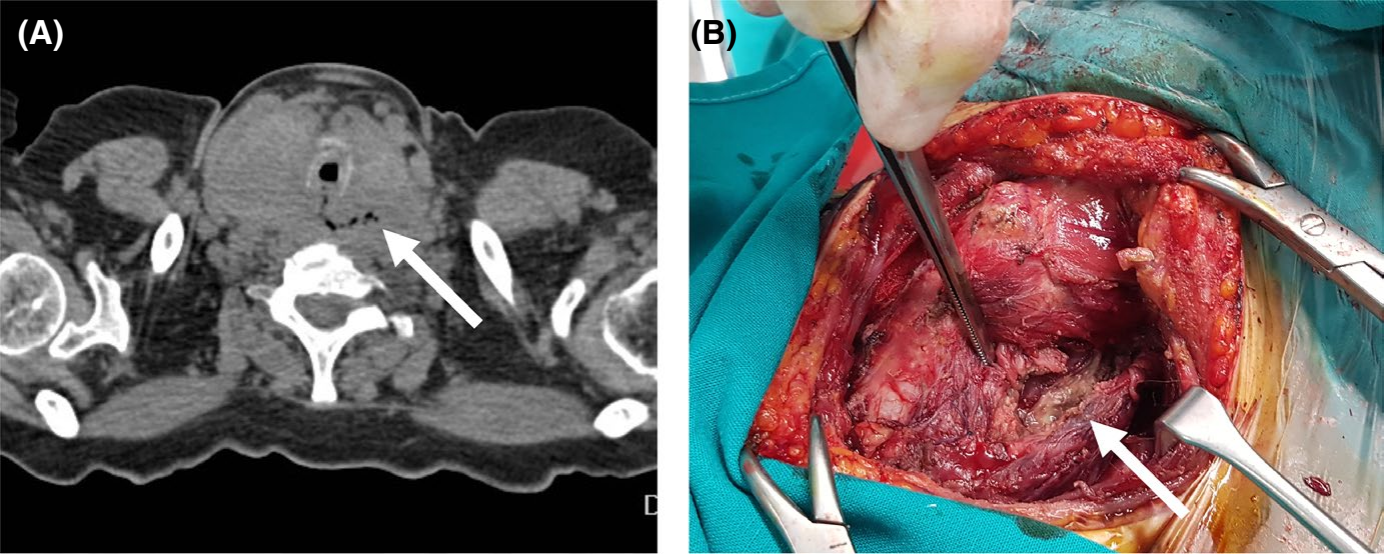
A, CT scan showing substernal goiter, trachea compression, and air bubbles surrounding the esophagus (white arrow). B, Intraoperative image showing perforation of the esophagus due to the thyroid lymphoma (white arrow)
